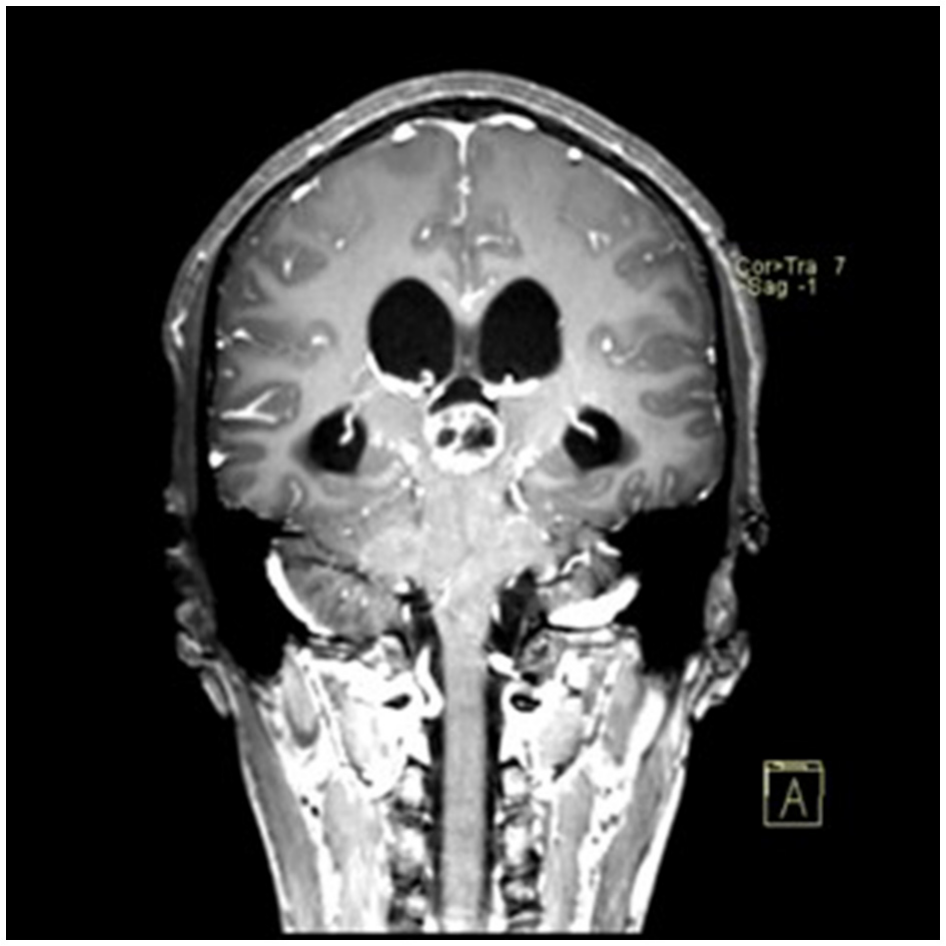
Preoperative contrast-enhanced MRI (coronal view) showing the pineal region tumor.
